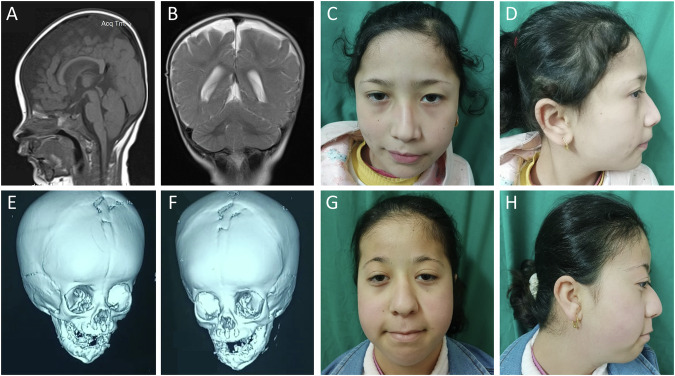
Craniofacial features of both patients, brain imaging of patient 1, and 3D CT skull of patient 2. Sagittal T_1_-weighted (A) and coronal T_2_-weighted (B) images of the brain of patient 1 at the age of 8 months showed no abnormalities. The cerebellar vermis and hemispheres had a normal appearance. C and D Patient 1 had high forehead, flat occiput, facial asymmetry, thin lateral and medial flaring of eyebrows, hypoplasia of the left upper eyelid, left epicanthic fold, upslanted palpebral fissures, deviated nasal septum with overhanging nasal tip, deep philtrum, downturned corners of the mouth, and multiple facial nevi. E and F 3D CT skull of patient 2 at the age of 2 months displayed premature fusion of the upper halves of coronal sutures and metopic sutures. G and H Patient 2 had high forehead, facial asymmetry, flat occiput, lateral thinning of the eyebrows, bilateral ptosis, downslanted palpebral fissures, depressed nasal bridge, deviated nasal septum, long philtrum, downturned corners of the mouth, thin upper lip, retrognathia, and multiple facial nevi
